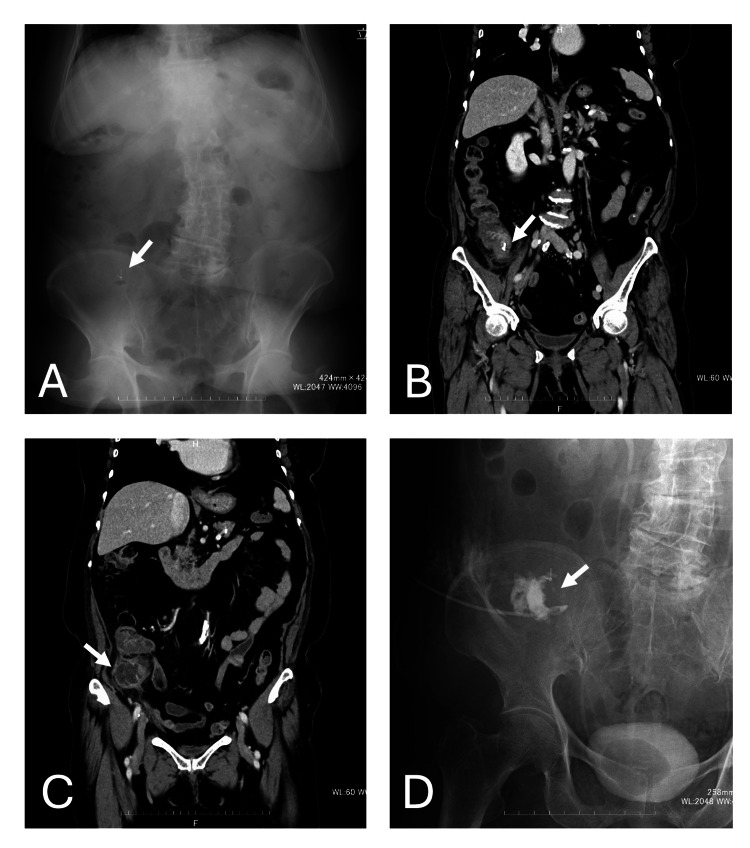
Imaging findings at admission and percutaneous abscess drainage. (A) Abdominal radiograph demonstrating a metallic foreign body (arrow) in the right lower abdomen, suspected to be an endoscopic clip. (B, C) Contrast-enhanced computed tomography showing a periappendiceal abscess adjacent to the cecum and a high-density foreign body near the base of the appendix (arrow), consistent with an endoscopic clip. (D) Percutaneous abscess drainage performed under ultrasound and fluoroscopic guidance. A 7-Fr pigtail catheter (arrow) was placed within the abscess cavity.
